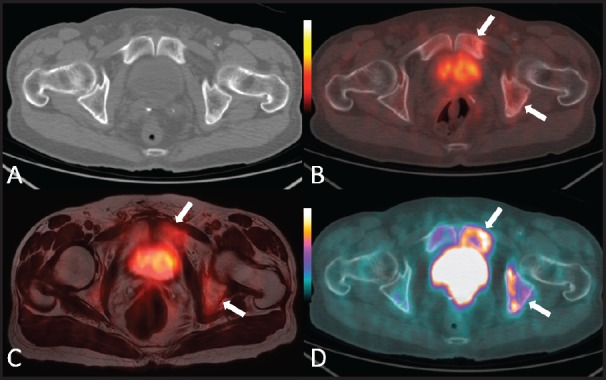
An 80-year-old man with bone metastases involving the left ischiopubic branch. The bone lesions are not detectable on the axial CT image with bone window (A) The ^18^F–choline–PET/CT (B) and the ^18^F–choline–PET/MRI fused (C) axial images show areas of moderate tracer accumulation in the left ischiopubic branch (arrows). The presence of metastatic bone involvement was further confirmed by means of ^18^F–fluoride–PET/CT scan (D) Images obtained with Quanta Prostate, Camelot Biomedical Systems s.r.l., Genoa, Italy.
